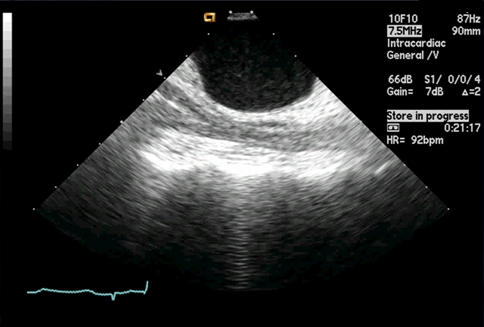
Linear phased-array intracardiac ultrasound image obtained from the right atrium of the posterior left atrial wall and adjacent to the esophagus. The outline of the esophagus can be seen just posterior to the left atrium at this site. Separating these two structures is the oblique sinus or the pericardium. If effusion or tissue changes (increased echogenicity) are noted in the oblique sinus, energy delivery must be stopped and ablation at another site or cryoablation should be considered.
